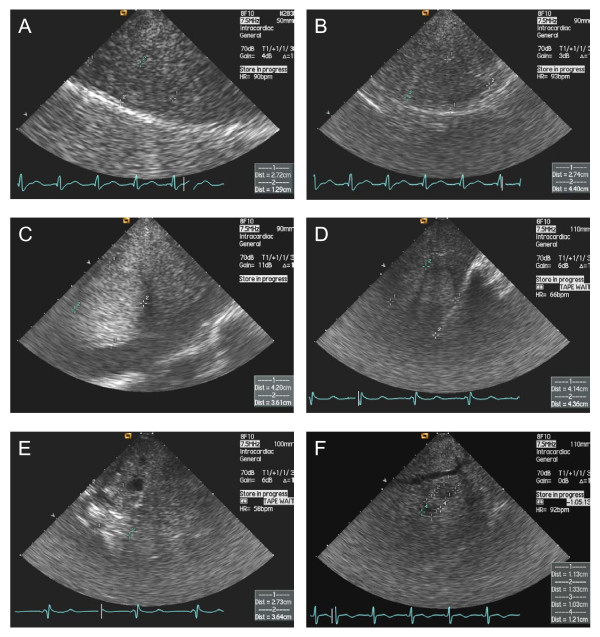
Examples of focal lesions identified by ICE in patients with liver cirrhosis. Without contrast medium for ultrasound, these tumors, which were highly suspect for hepatocellular carcinoma, could not be differentiated from regenerative nodules. The ultrasound device was introduced into the right or middle hepatic vein and the parenchyma surrounding the vessel was inspected over 360 degrees by turning the ultrasound catheter clock- and anti-clockwise. The ultrasound pattern of these lesions was homogeneous (A, B and C) or heterogeneous (D, E and F). No vascular invasion was identified in this study.
